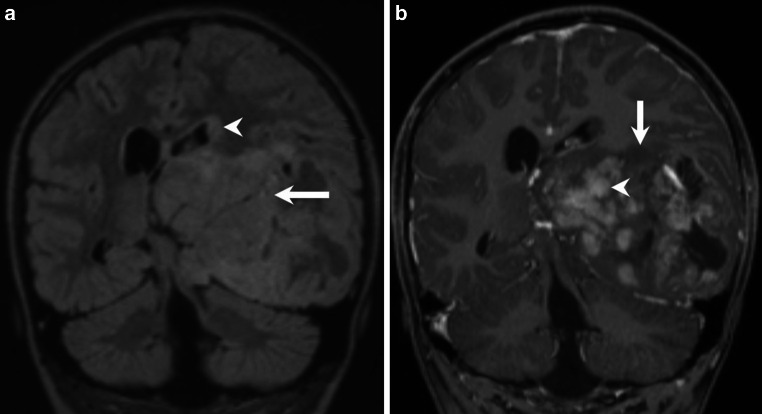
Coronal fluid-attenuated inversion recovery (FLAIR) images (a) show a huge space-occupying lesion of the left temporal lobe (*arrow*) causing a distinct midline shift to the right. A subependymal hypersignal can be seen at the level of the cella media of the left lateral ventricle (*arrowhead*). On coronal T1 weighted images after administration of gadolinium (b) the lesions shows a heterogeneous pattern of enhancement with a large number of well-delineated portions showing homogeneous enhancement (*arrowhead*) and areas with virtually no contrast enhancement (*arrow*)
